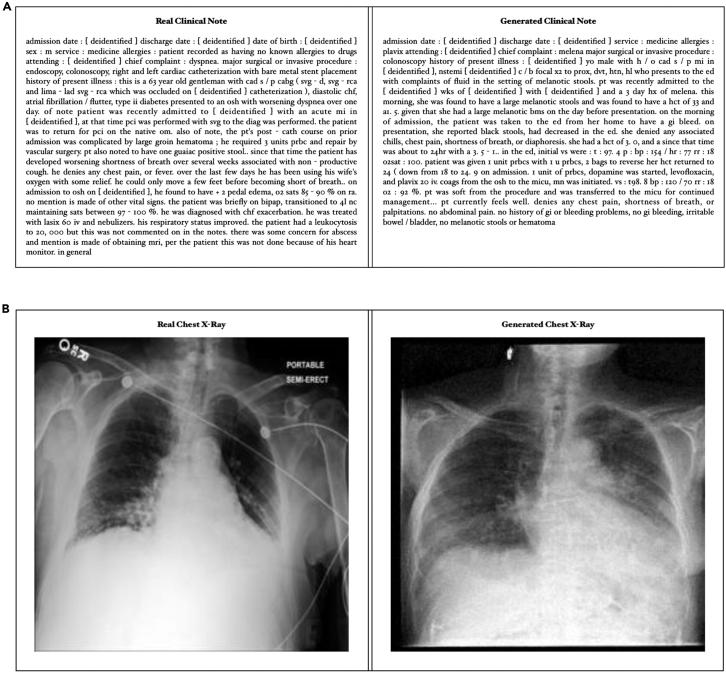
MediSim-generated samples of complex medical data modalities (Top) A synthetically generated clinical note and the real clinical note from the same visit. (Bottom) A synthetically generated chest X-ray and the real image from the same visit. While the generative models act as a sample approach to demonstrate support within the MediSim framework rather than fully optimized models, they still show the ability to approximate the format and content of the real data and also incorporate the conditioning content, such as the presence of a colonoscopy in the note and lung opacity in the image.
